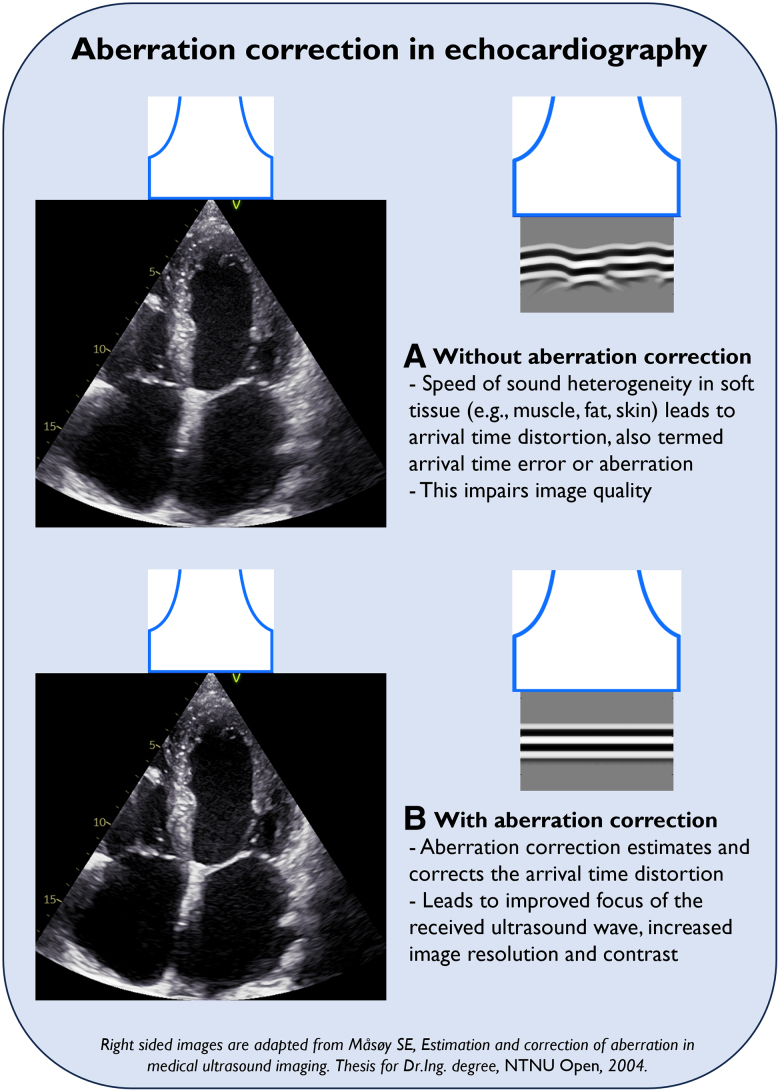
Aberration correction in echocardiography. The figure displays an apical 4-chamber view image from the same recording with conventional processing (*A*) and with AC (*B*). In this example, AC improved the definition of the endocardial border, perhaps most pronounced in the left atrium. Moreover, anatomical details of the mitral valve and the subvalvular apparatus appear sharper with less lateral ‘smearing’.
